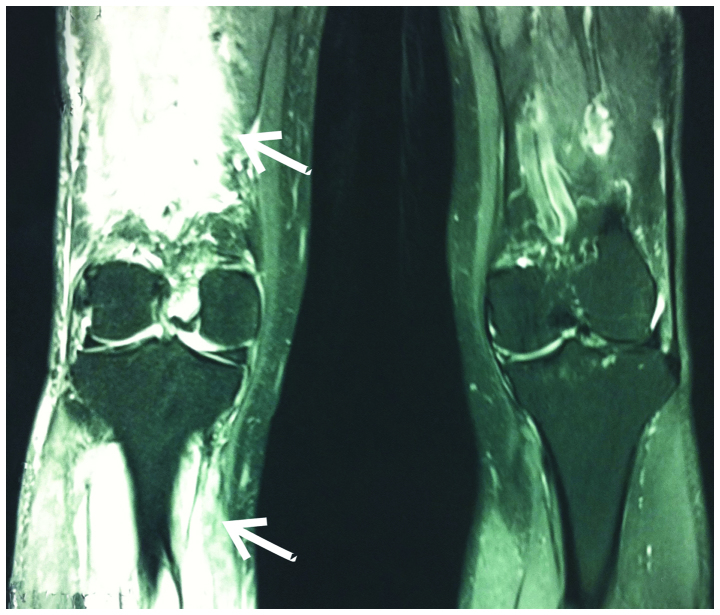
Features of the enhanced T1-weighted magnetic resonance imaging scans. Coronal section revealed a bulky uniform distension of skeletal muscles, which was hyperintense relative to normal muscle.
